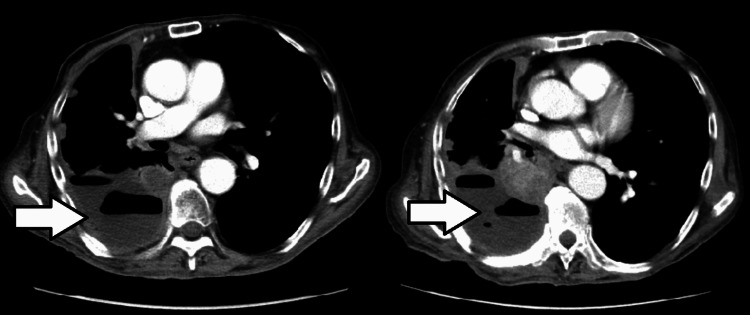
CT scan of the chest (left) showing pleural effusion (white arrow), unchanged from the prior CT scan of the chest (right).
